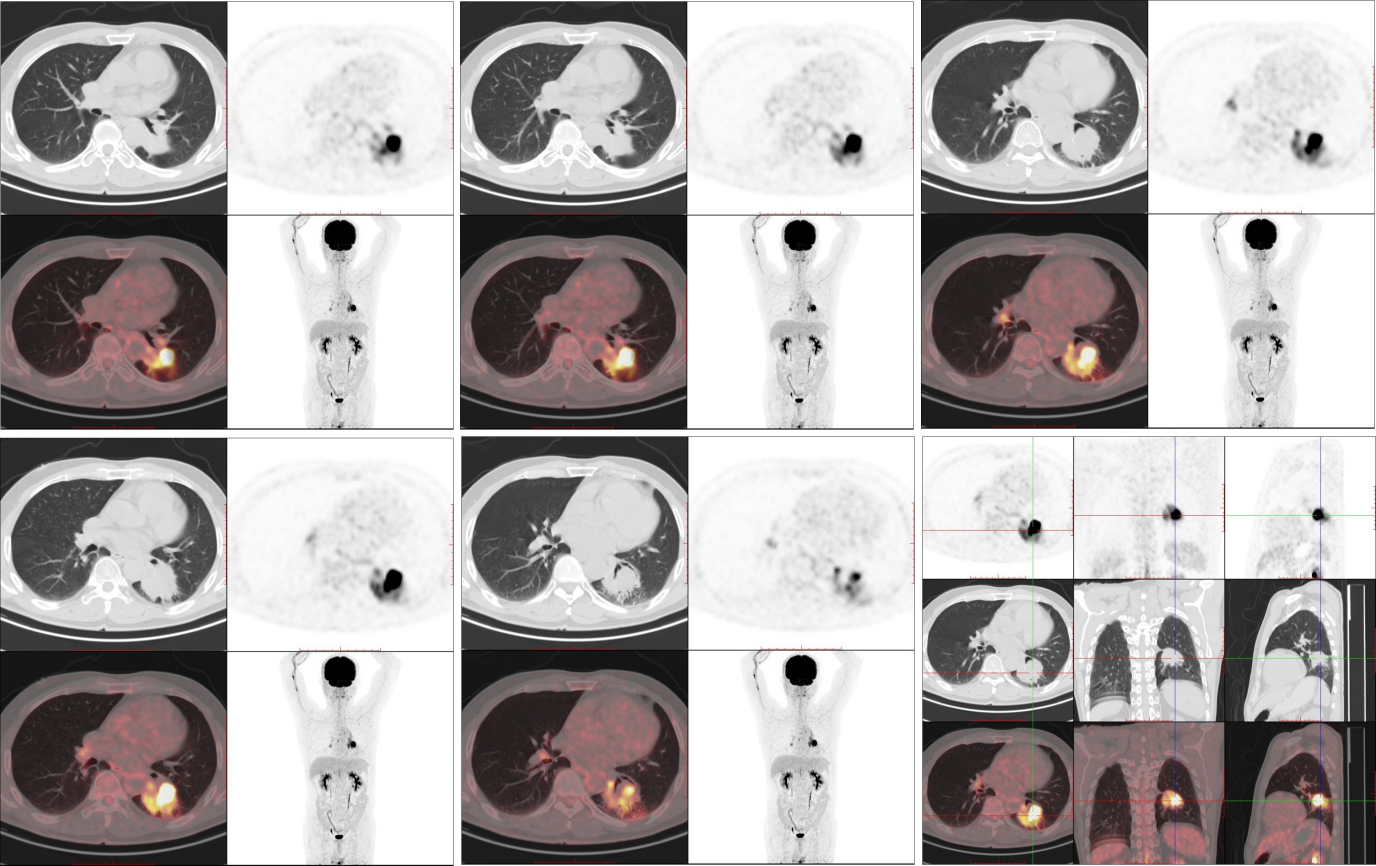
The 18F-FDG PET/CT examination (August 3, 2022). 18-FDG PET/CT shows robust 18-FDG uptake in the left lung mass (SUVmax: 27.998) with a size of 45 × 41 mm; no distant metastasis can be observed.
